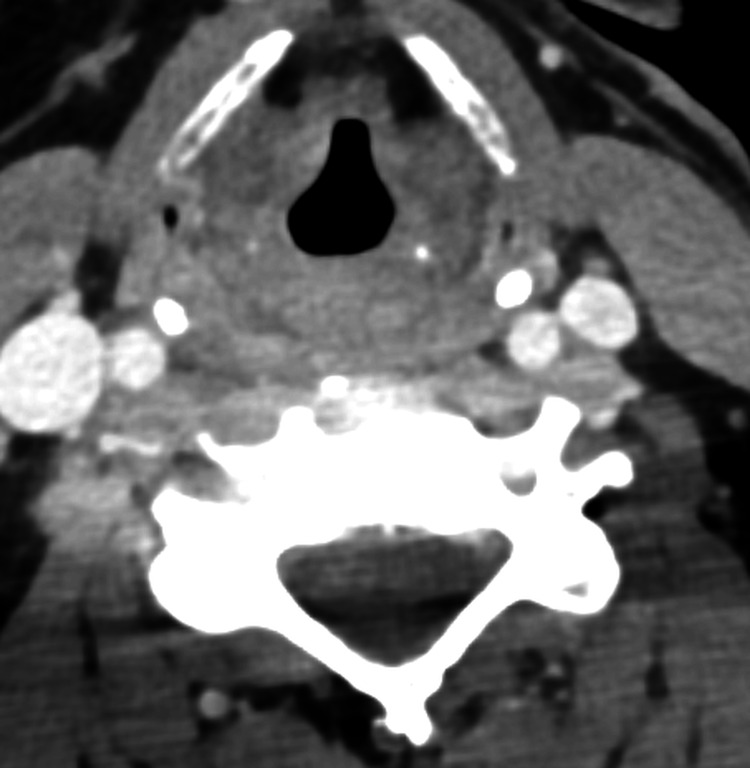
CT of the neck with contrast, which revealed edema involving the true and false vocal folds, aryepiglottic folds, and paraglottic fat
